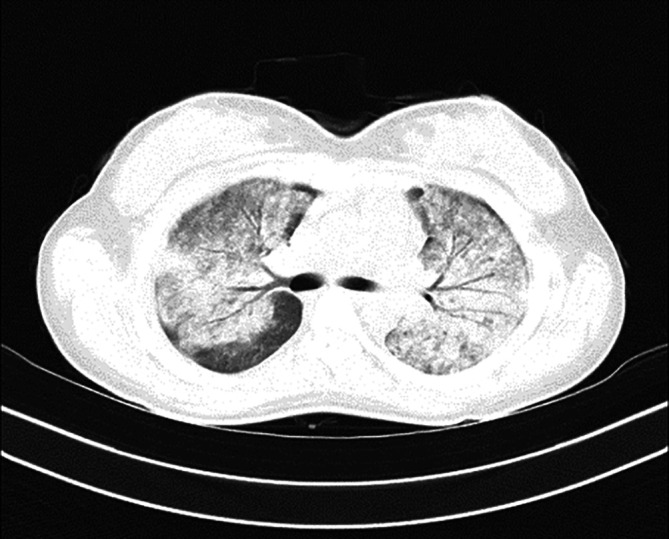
On admission, HRCT of the thorax shows bilateral extensive diffuse areas of ground glass opacification with superimposed patchy consolidation; with a CT severity score of 21/25. These findings were consistent with the characteristic imaging manifestations of scrub typhus-associated ARDS.
